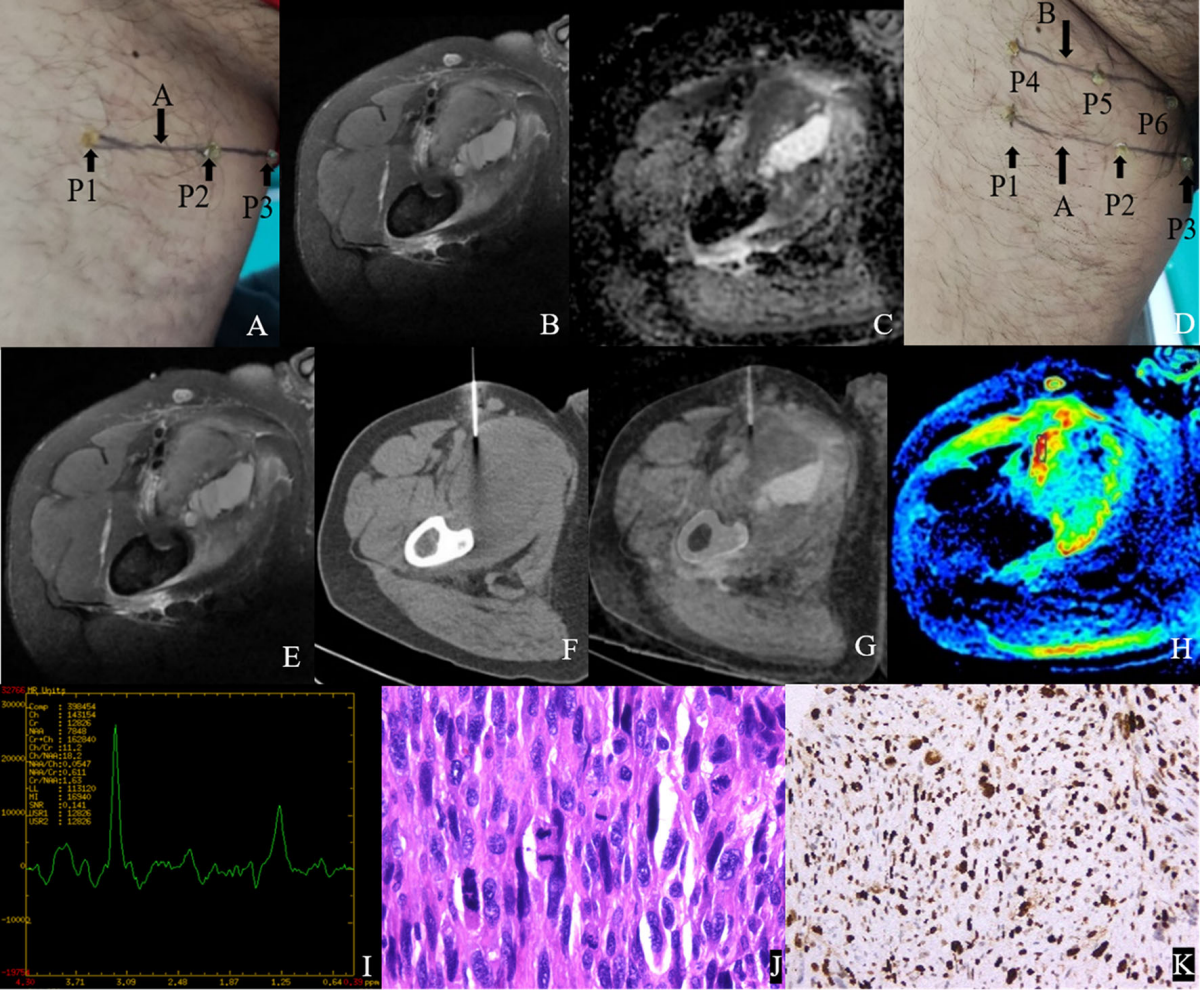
Male, 47Y, Pleomorphic leiomyosarcoma of the right thigh, G3. (A) Body surface positioning marks before MRI examination; (B, C) Targeted slice, chosen by Axial T2WI-FS and ADC image; (D) Mark the targeted slice on body surface; (E) A second time Axial T2WI-FS scan to confirm the target slice; (F) CT guided biopsy at target slice; (G) CT-MRI image fusion at target slice; (H) Draw ROI to obtain DWI quantitative parameters, ADCmin: 0.725×10–^3^ mm^2^/s, ADCmean: 0.929×10–^3^ mm^2^/s; (I)
